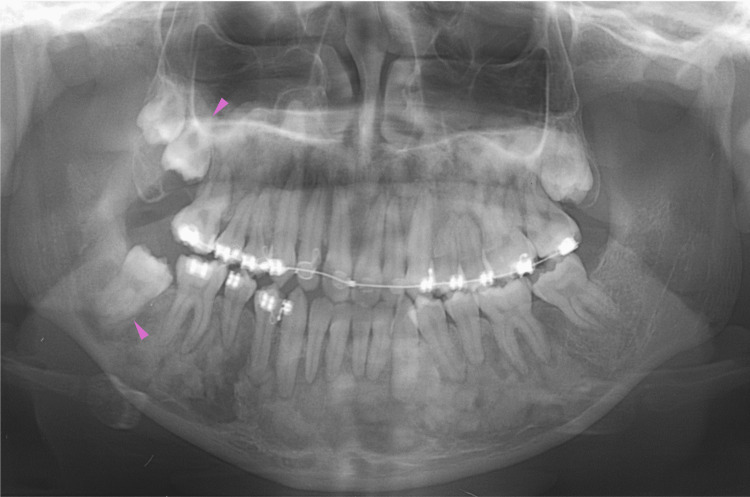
Maxillofacial radiograph at age 17 Purple arrowheads indicate bony adhesions (ankylosis) in the right upper and lower second molars
